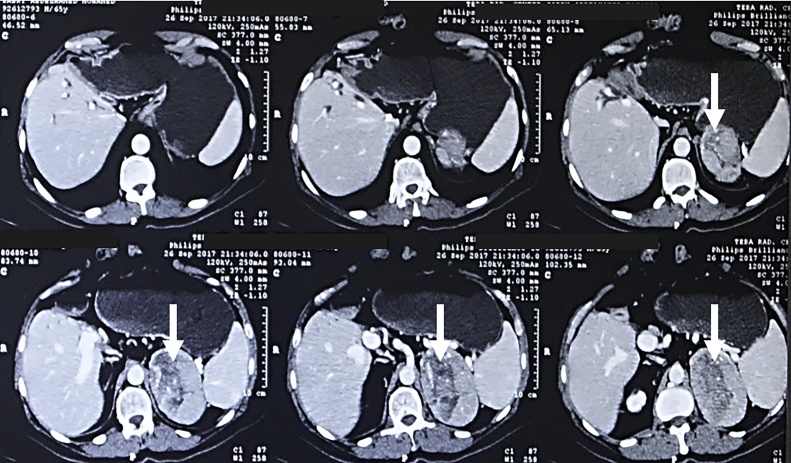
Abdominal computed tomography 5 years after liver transplantation showing large left adrenal mass (white arrow).
